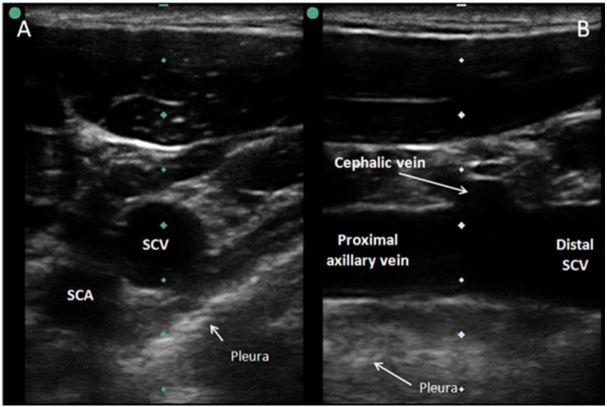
A) Short axis view of subclavian vein using ultrasound vascular probe. B) Long axis view of subclavian vein using ultrasound vascular probe. *SCV*, subclavian vein; *SCA*, subclavian artery
